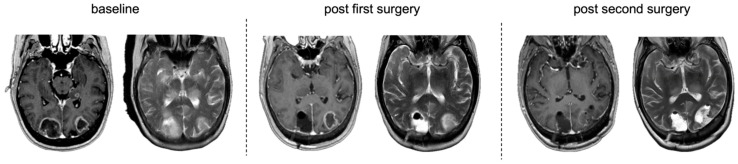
MRI from a representative case with multifocal approaches and supramaximal resection after two-stage surgery. From left to right. T1-CE and T2-nCE baseline to post second surgery. Postoperative MRI demonstrating T1-CE as well as T2-nCE residual tumour in the left occipital lobe. MRI after the second surgery shows no residual tumour.
